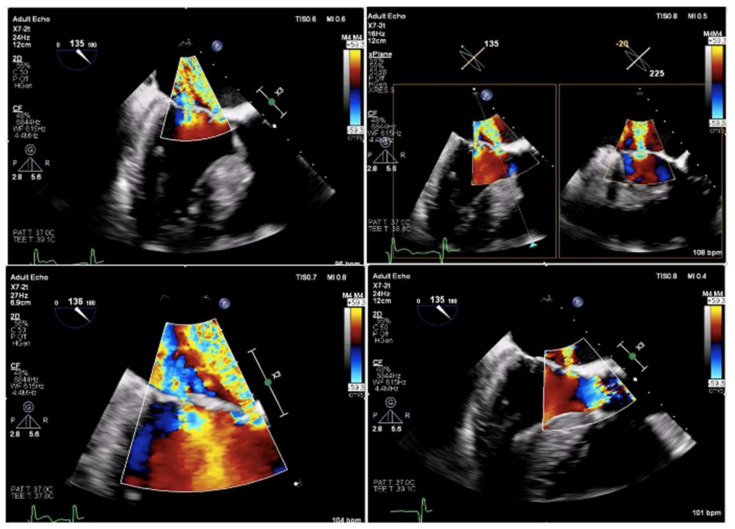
Intraoperative transesophageal echocardiography findings just after deployment of the transcatheter aortic valve showing anterior mitral leaflet perforation that caused an increase in the degree of MR.
